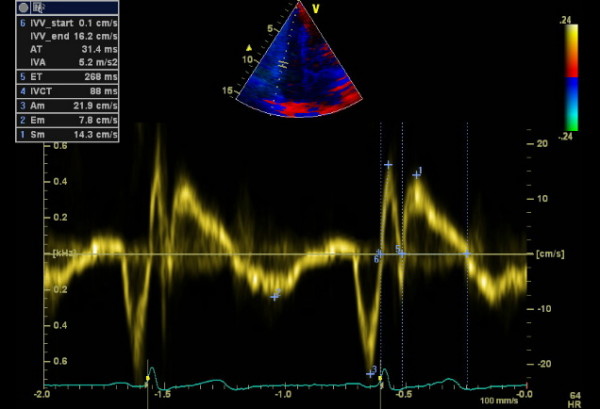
Pulsed wave tissue Doppler imaging of the RV free wall of a control subject. 1: peak myocardial systolic velocity (Sm), 2: peak early diastolic velocity (Em), 3: peak late diastolic velocity (Am) 4: isovolumetric contraction time (IVCT), 5: ejection time (ET), 6: peak myocardial isovolumetric contraction velocity (IVV), acceleration time (AT), isovolumetric acceleration (IVA).
